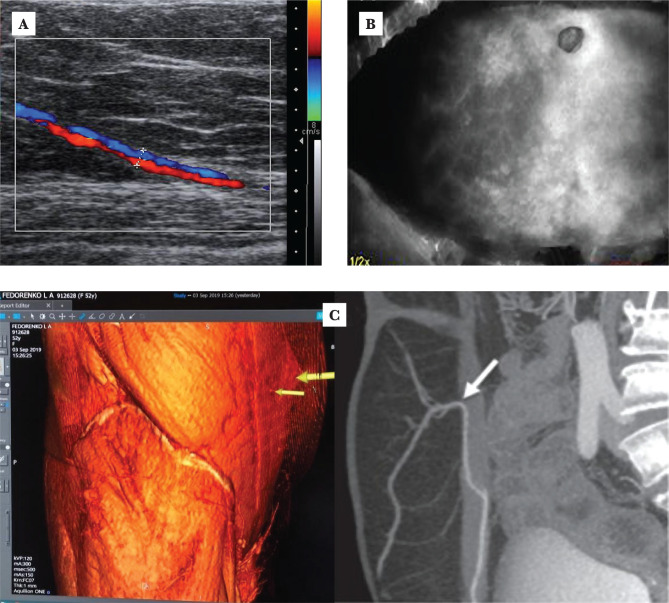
Preoperational diagnostics of location and parameters of major perforating vessels: A – ultrasonic duplex scanning; B – fluoptics fluobeam; C – computer tomography with angiography.
